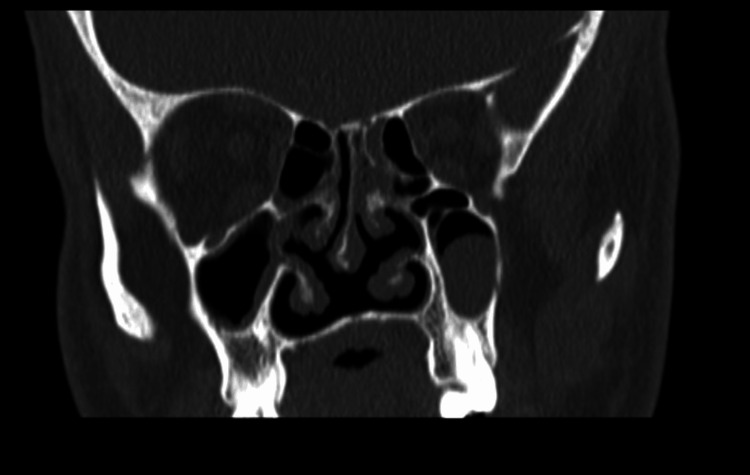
CT scan of the paranasal sinus, coronal view, showing a right-sided septal spur
